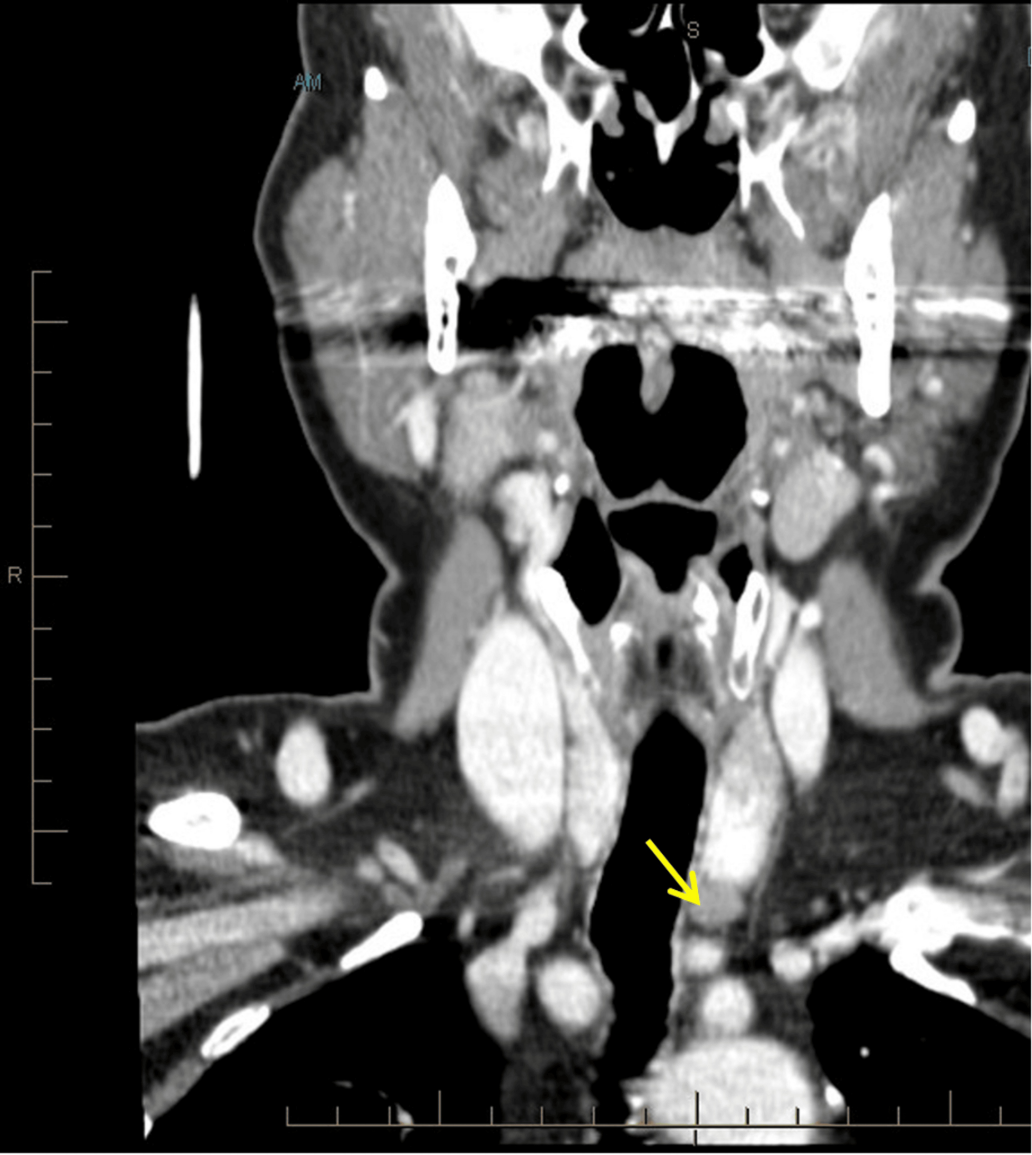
Neck CT scan with contrast. The yellow arrow indicates the schwannoma, measuring 1.1 cm.
